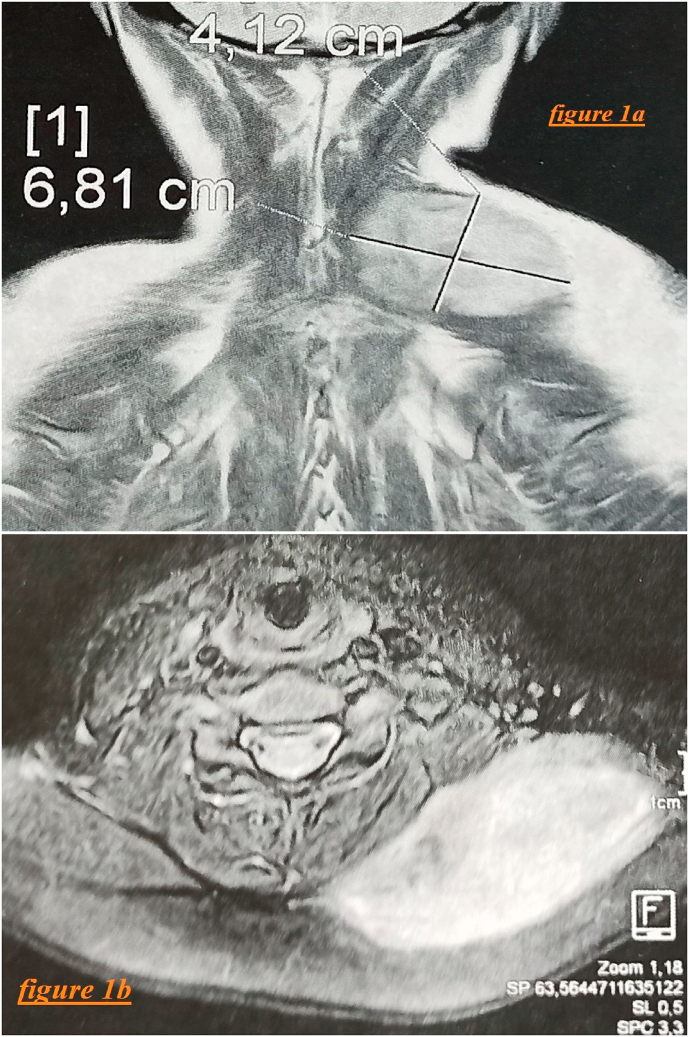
(1a and 1b): Cervical MRI showing mass of the left posterior cervical soft parts, at the expense of the trapezium muscle, measuring 41 × 68 × 81 mm.
